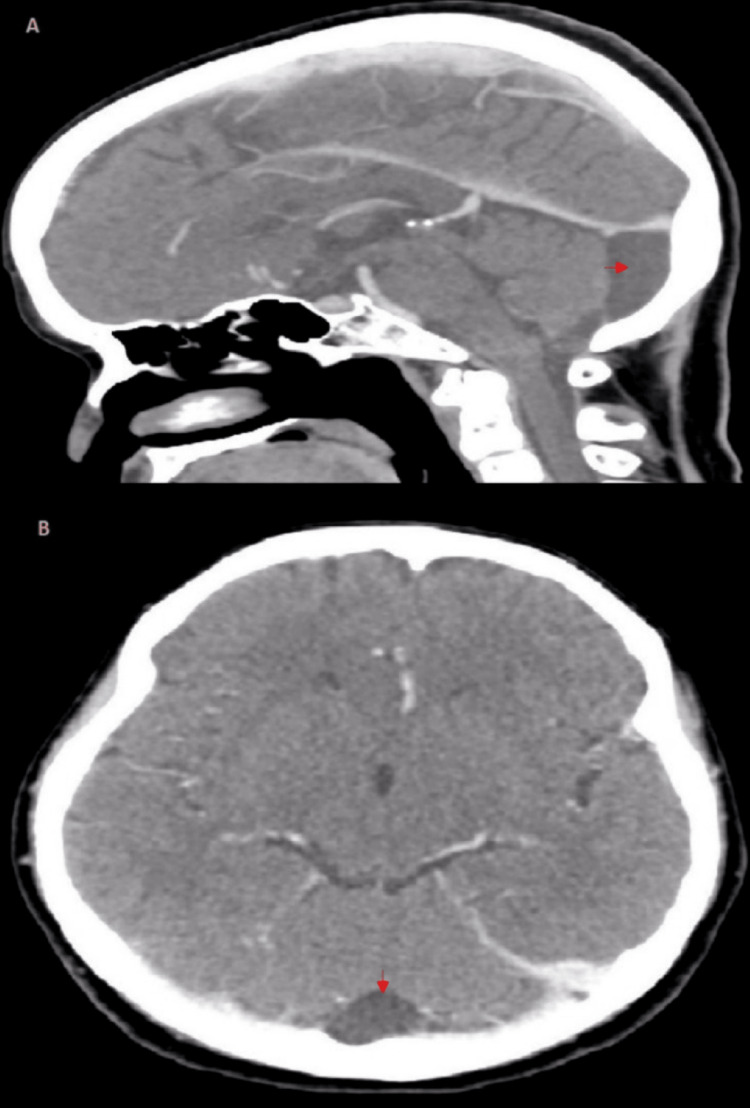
Sagittal (A) and axial (B) view of the contrasted CT images of the brain shows fluid density lesion in the posterior fossa region (red arrow).
